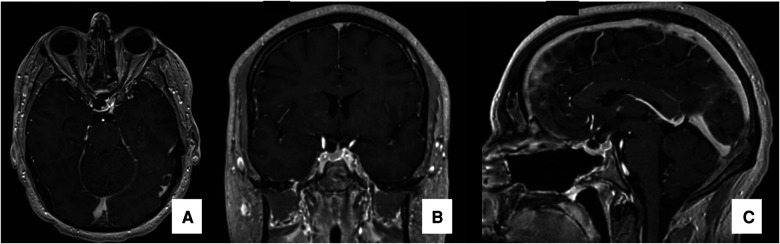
Postoperative MRI postoperative MRI in T1WI images with gadolinium demonstrating gross-total resection of the tumor: axial (A), coronal (B) and sagittal (C) views.
